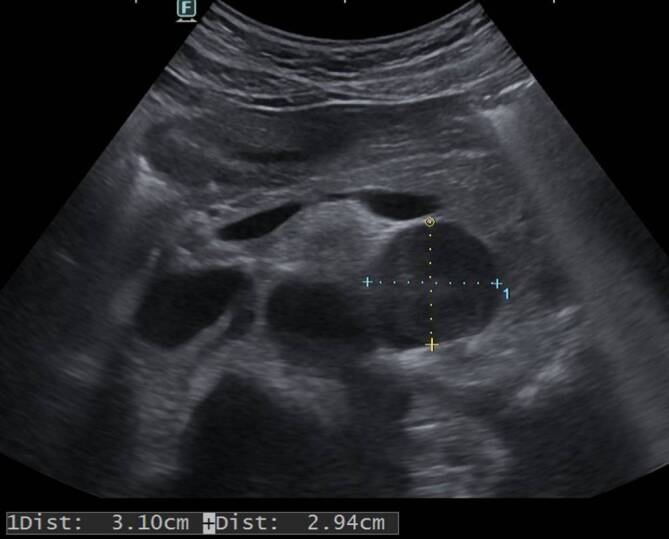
Sonographischer Transversalschnitt des Oberbauchs mit Darstellung einer ca. 3 × 3 cm großen inhomogenen Raumforderung paraaortal links
